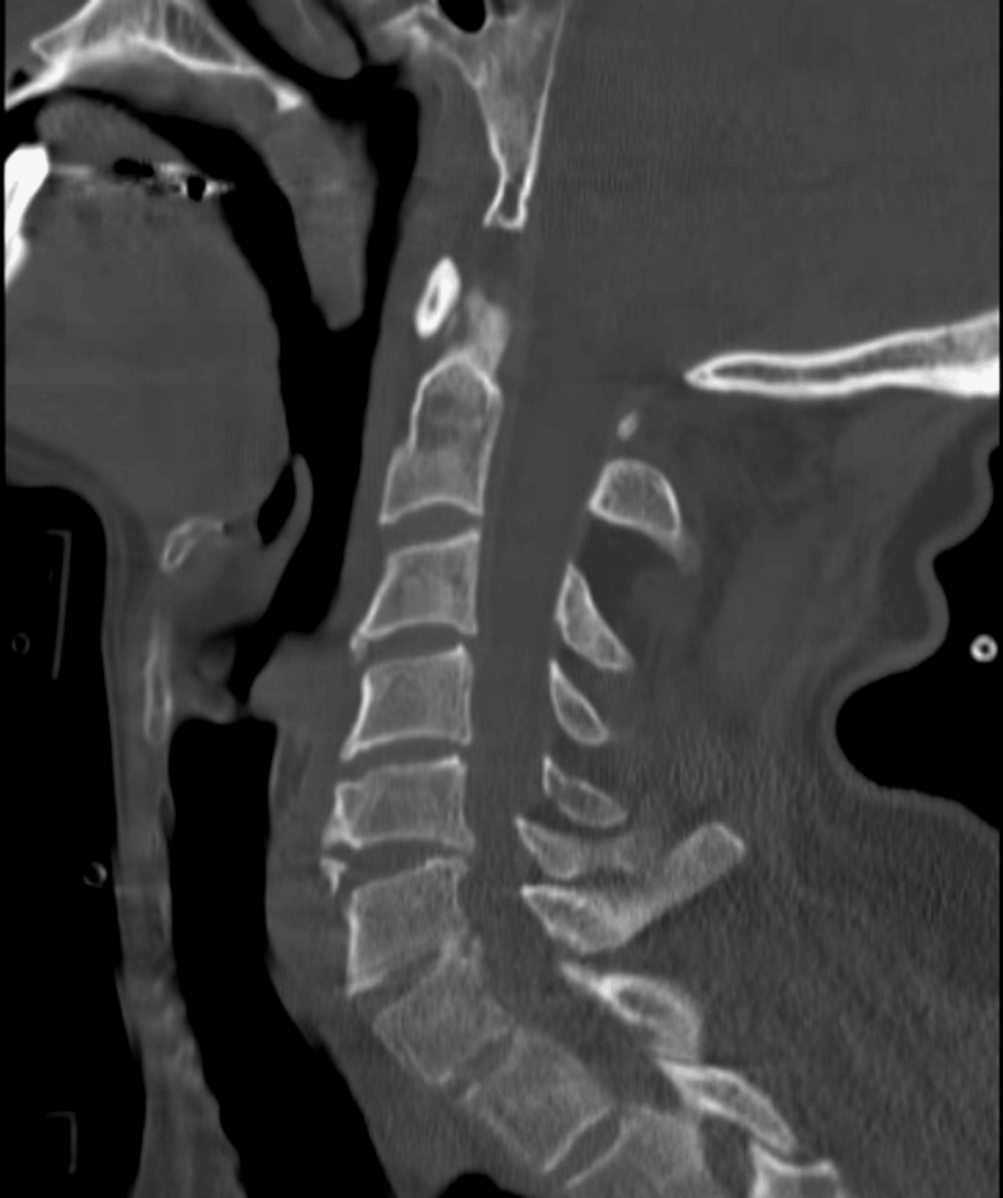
Sagittal view of the CT cervical spine, read as normal with chronic degenerative changes
